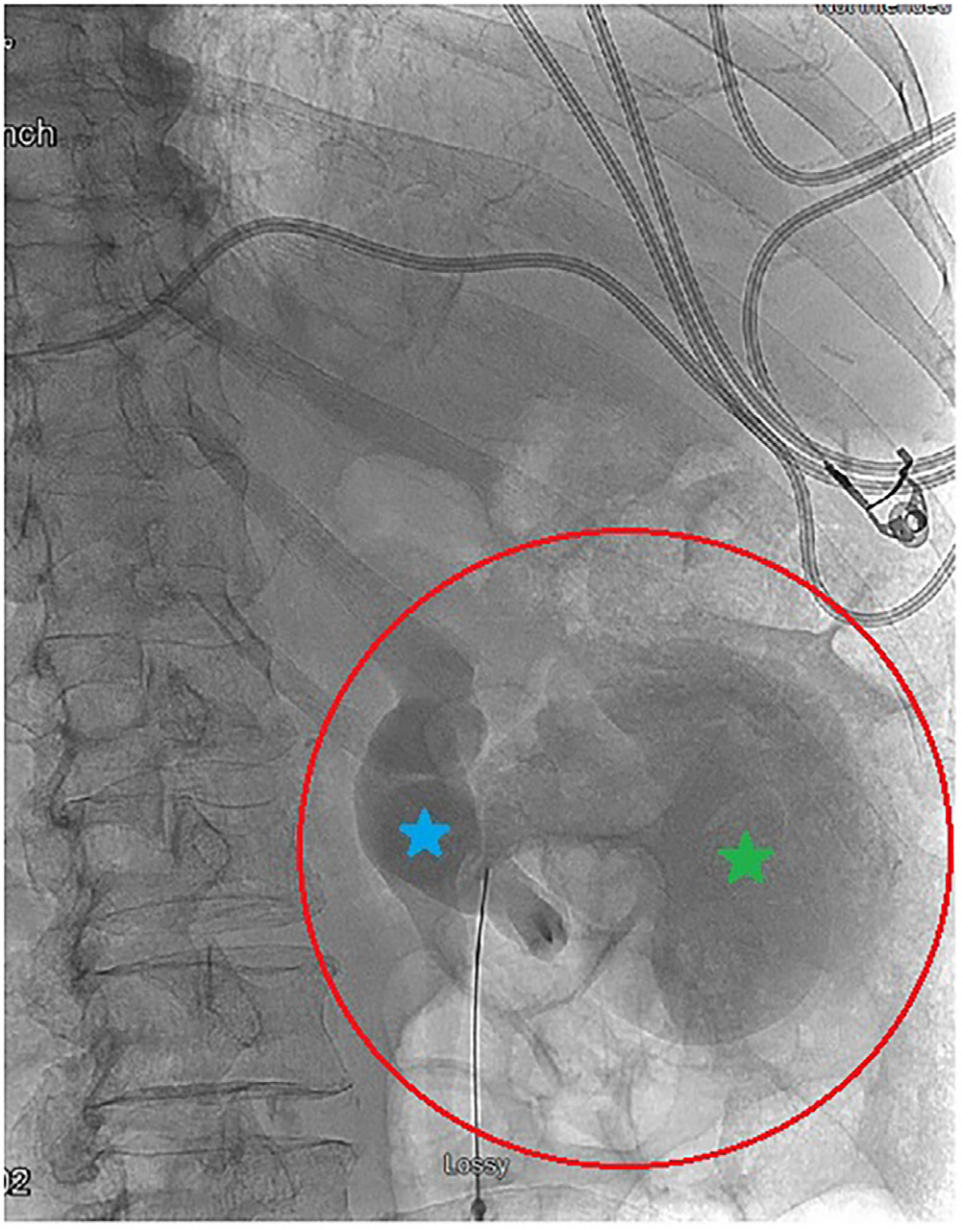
Fluoroscopic imaging of the contrast collection in the right renal cyst. Contrast from the earlier CT imaging that day has collected in the renal cyst. This implies a communication between the renal cyst and the collecting system’s renal pelvis (blue).
